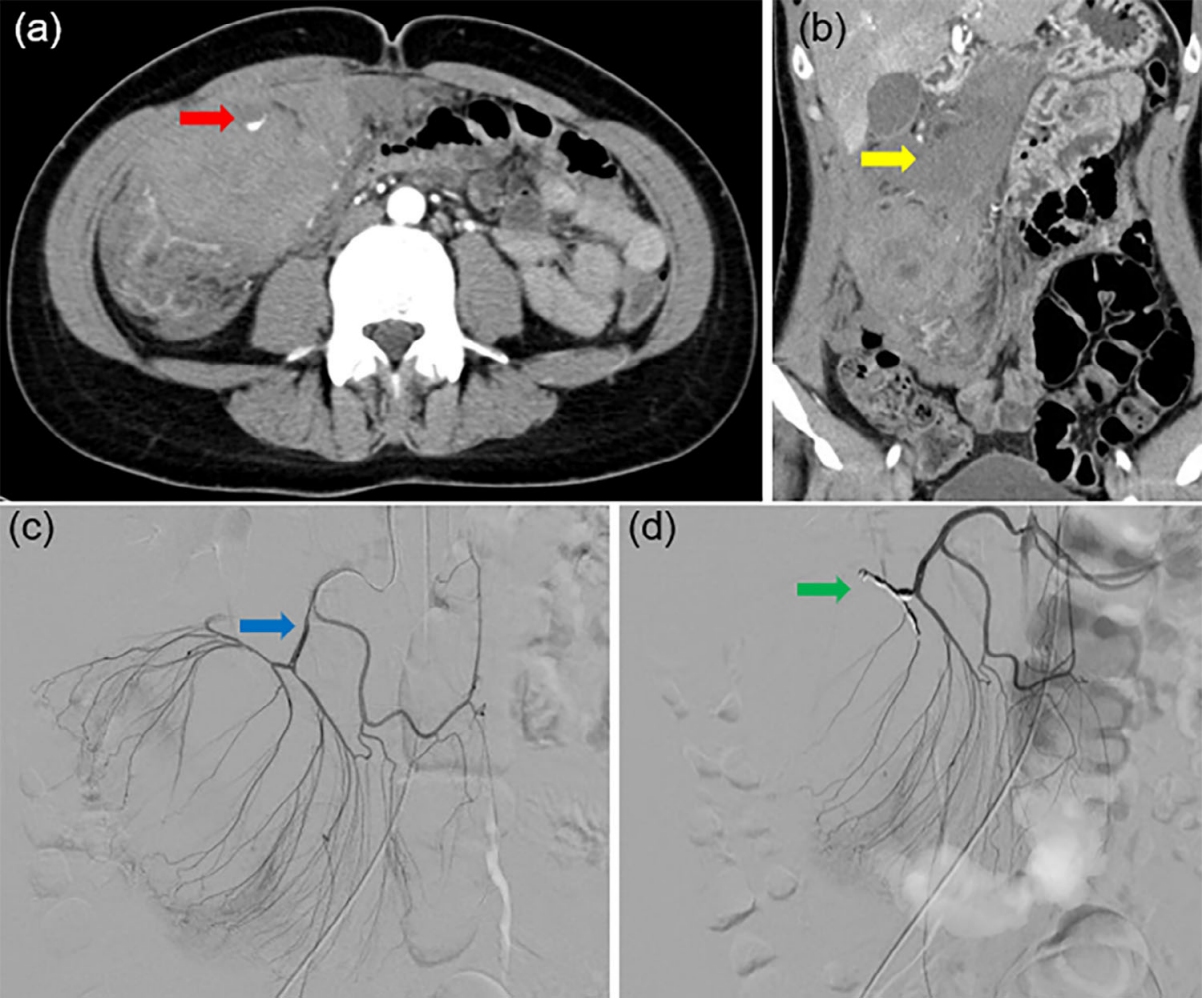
Contrast‐enhanced computed tomography and transcatheter embolization: (a) a 70 mm, hematoma in the transverse colon with contrast extravasation (red arrow), the outer layer of the bowel in the hematoma‐forming region was not discernible; (b) coronal view of high attenuation ascites (yellow arrow); (c) middle colic arteriography couldn't show the bleeding point, but it was considered the vascular supply route (blue arrow); (d) coil embolization was performed on a branch of the middle colic artery for the purpose of preventing rebleeding (green arrow).
